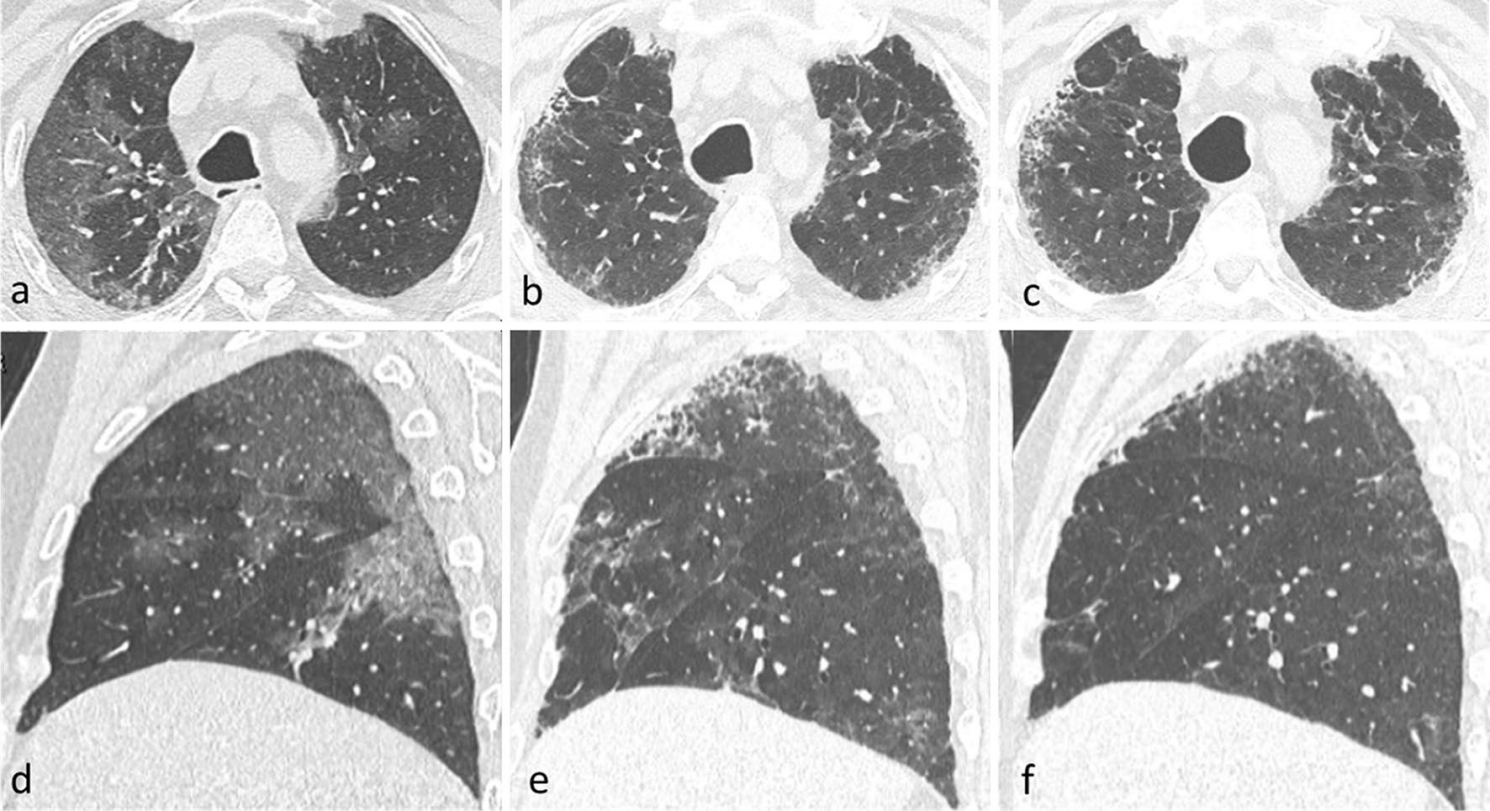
Disease evolution pattern consistent with fibrotic abnormalities (Non-Specific Interstitial Pneumonia, NSIP pattern). Axial (a–c) and sagittal (d–f) CT images showing patchy ground glass opacities at baseline (a–d), subtle residual ground-glass opacities, and appearance of subpleural reticulation and traction bronchiectasis in upper lobes at 2–3 months (b–e) and at 6–7 months (c–f).
